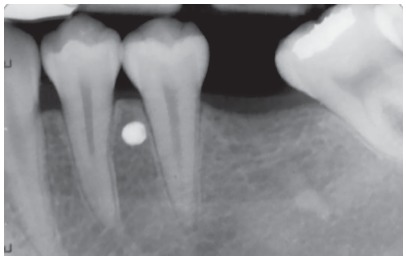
Periapical radiograph after mini-implant placement.
